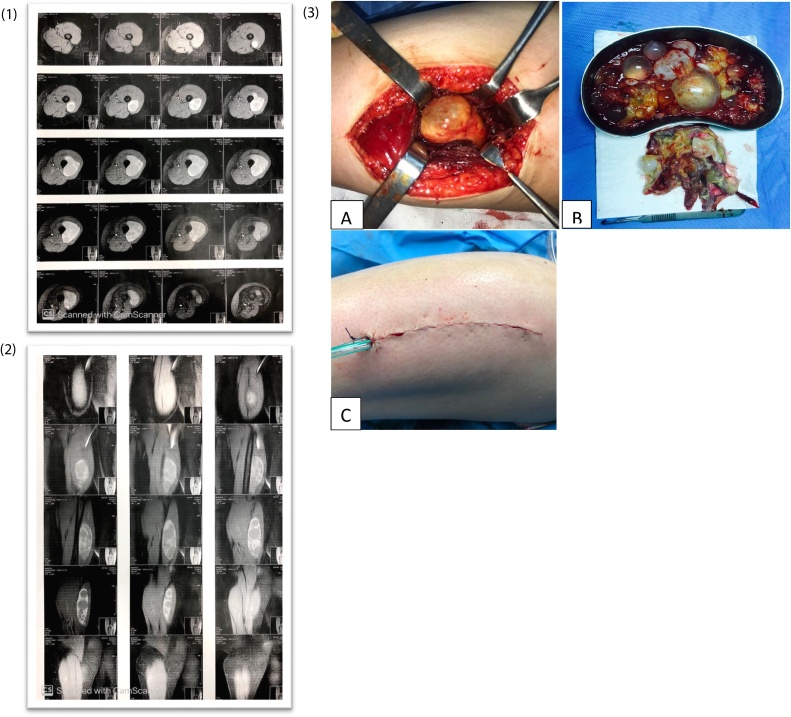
1. Ultrasonography of thigh showed 15 cm × 5 cm solid-cystic with multiple well defined cystic lesion. 2. The MRI featured a large multisystem lesion. 3. Photographs of lateral thigh show; A and B: Inner germinated layer of hydatid cyst. C: A drain fixed at the end of procedure.
